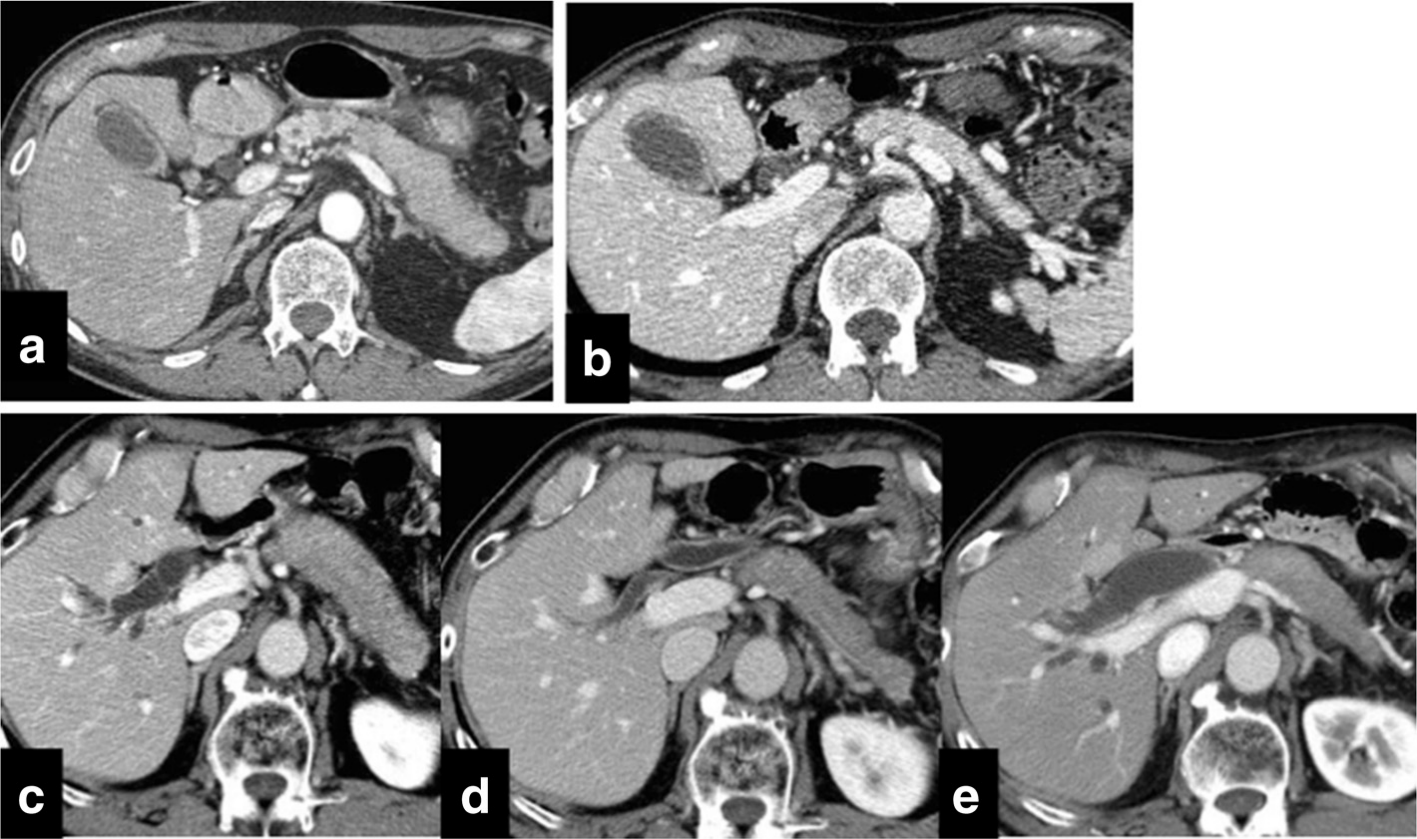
Abdominal computed tomography showing the pancreas in patients with autoimmune pancreatitis. The post-treatment pancreatic volume was 30.3 cm^3^, and the percent reduction in the pancreatic volume was 68.2 % in a patient who showed a favorable response without relapse before steroid therapy (a) and 2 weeks after starting steroid therapy (b). The pancreatic volume was not reduced in a patient who showed an unfavorable response with relapse. The post-treatment pancreatic volume was 51.6 cm^3^, and the percent reduction in the pancreatic volume was 42.3 % before steroid therapy (c) and after 2 months starting steroid therapy (d); at 47 months during relapse, dilatation of the biliary tree (e) was observed
